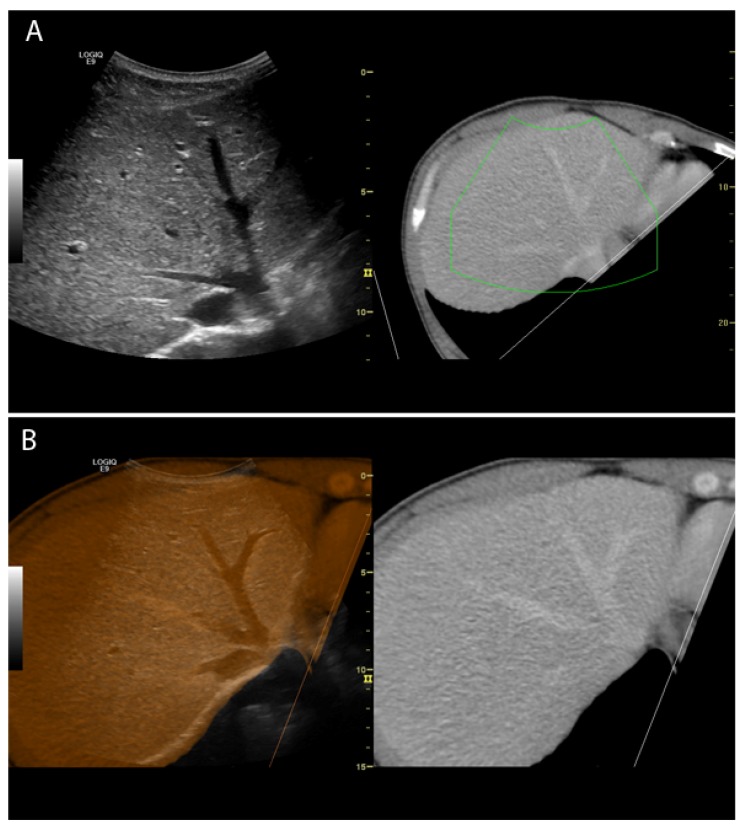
(A) Co-registered images of the liver shown side by side (ultrasound (US): left; computed tomography (CT): right); The green box indicates the scanning area (B) CT and US images of the liver overlaid (left) and corresponding CT-image (right).
